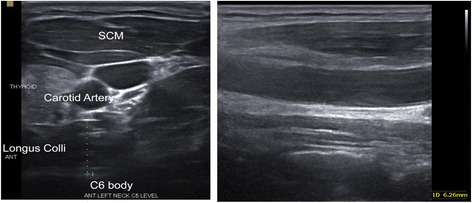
Ultrasound image of the longus colli at C6 in transverse view to the left behind the carotid artery, and to the right longitudinal where measurements were taken. The caliper is placed on the midpoint of the ventral surface of the C6 vertebral body and the interface between the Lcol and the pre-fascial tissue surrounding the carotid artery
